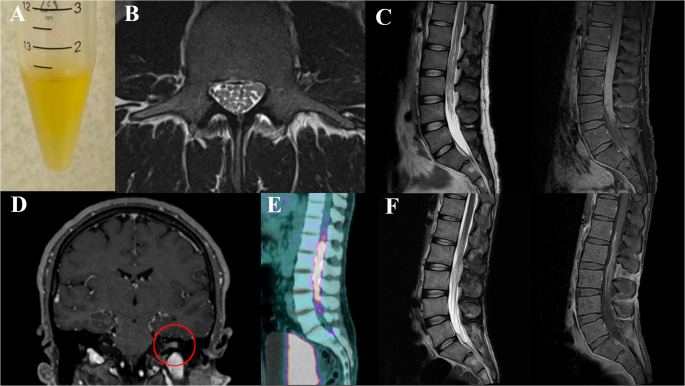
A Cerebrospinal fluid (CSF) appearance showing marked xanthochromia; B Pre-treatment axial T2-weighted MRI images; C Pre-treatment sagittal T2-weighted and contrast-enhanced fat-suppressed Dixon MRI images demonstrating marked thickening and clumping of the cauda equina nerve roots with associated enhancement; D Coronal reconstruction of a post-contrast three-dimensional T1-weighted fat-suppressed gradient-echo MRI sequence showing thickening and enhancement of the left facial–vestibulocochlear nerve complex; E 18F-FDG PET/CT demonstrating significant radiotracer uptake in the spinal cord; F Follow-up MRI performed after 3 months of therapy showing a significant reduction in the volume of the cauda equina nerve roots with decreased contrast enhancement
